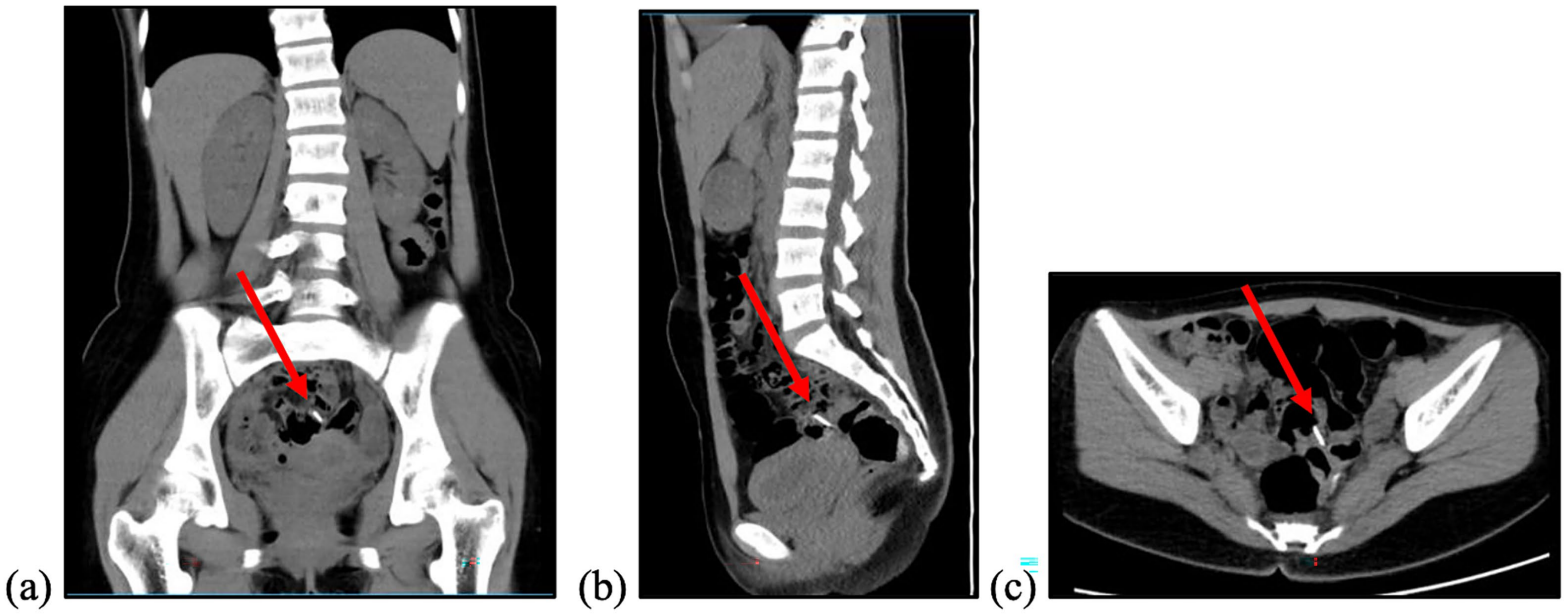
Abdominal CT Scan with contrast performed on Day 23 after ingestion showed a foreign body (red arrow) within the projection of the minor pelvis, within the ileum. (a) Coronal view; (b) sagittal view; (c) axial view.
